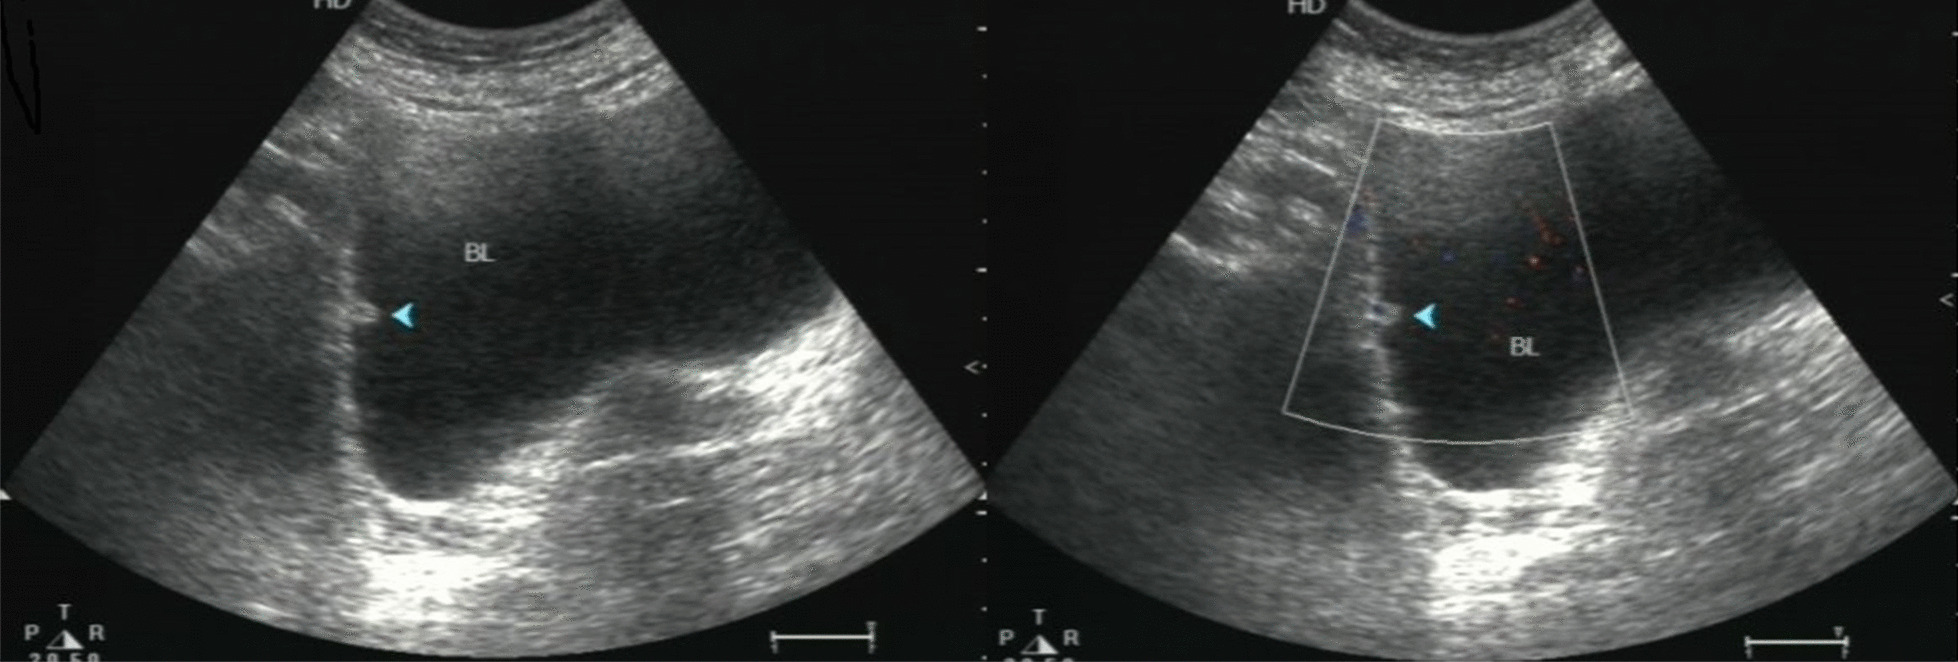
Bladder ultrasound indicated a homogeneous isoechoic polypoid mass with a maximum diameter of 6 mm at the right lateral wall of bladder
